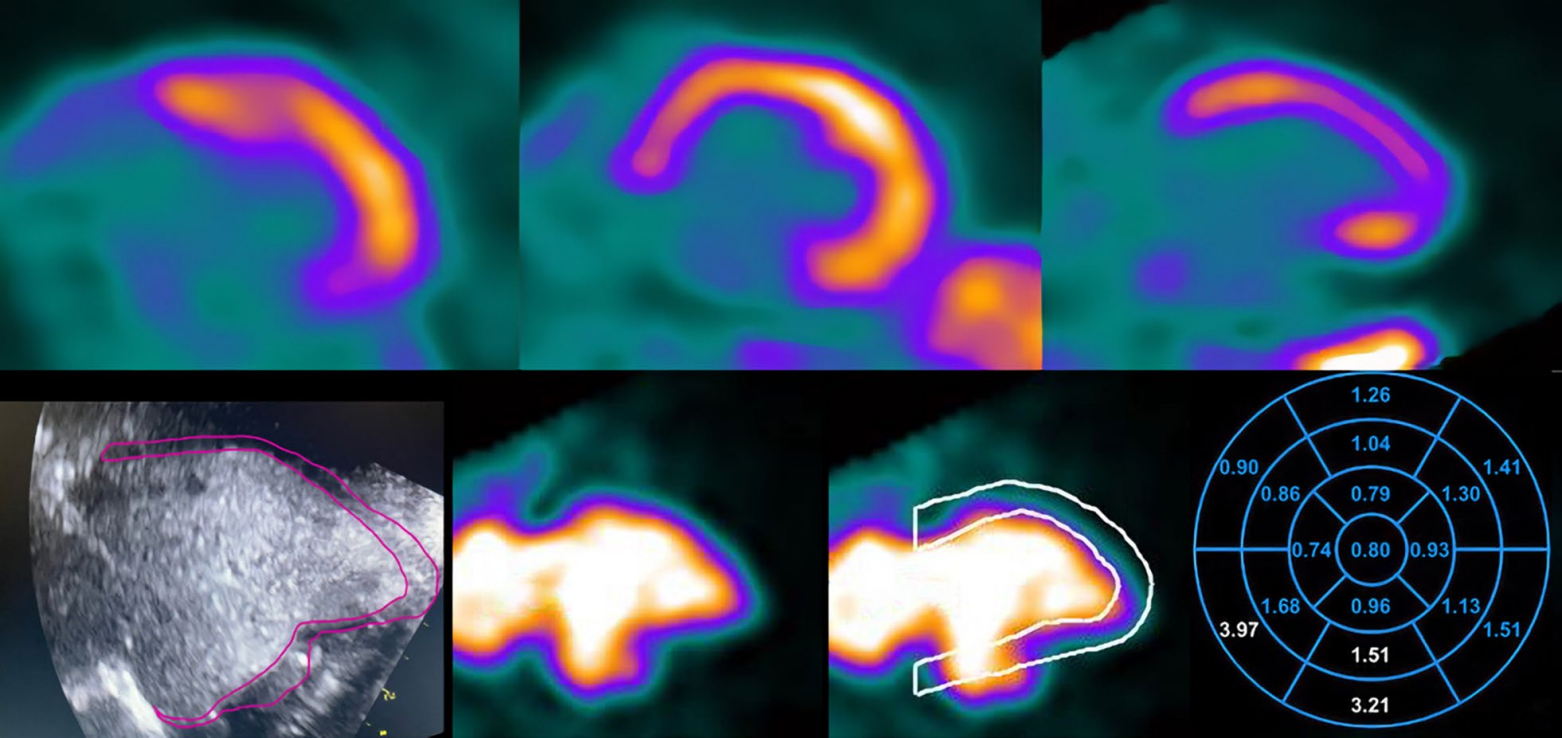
Inaccurate boundary selection. Due to the absence of myocardial uptake and LV aneurysmal segments within the transmural scar, the software package inaccurately identified myocardial boundaries, an error which could not be remedied manually with standard tools within the SWP. This erroneous boundary detection led to a “spillover effect,” yielding falsely elevated MBF values in the scar. Bottom left corner is an echocardiogram with the LV aneurysm outlined in pink
